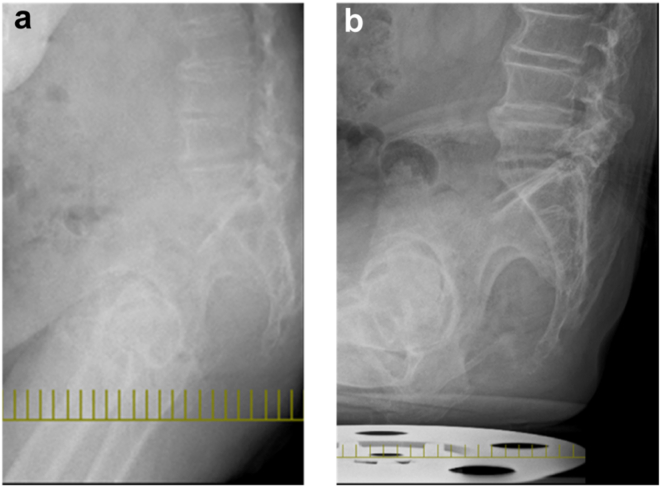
Lateral view of lumbar-pelvic radiograph. (a) SS measured at 11° in the standing position and (b) SS measured at 4° in the sitting position. Δ SS = 7°.
